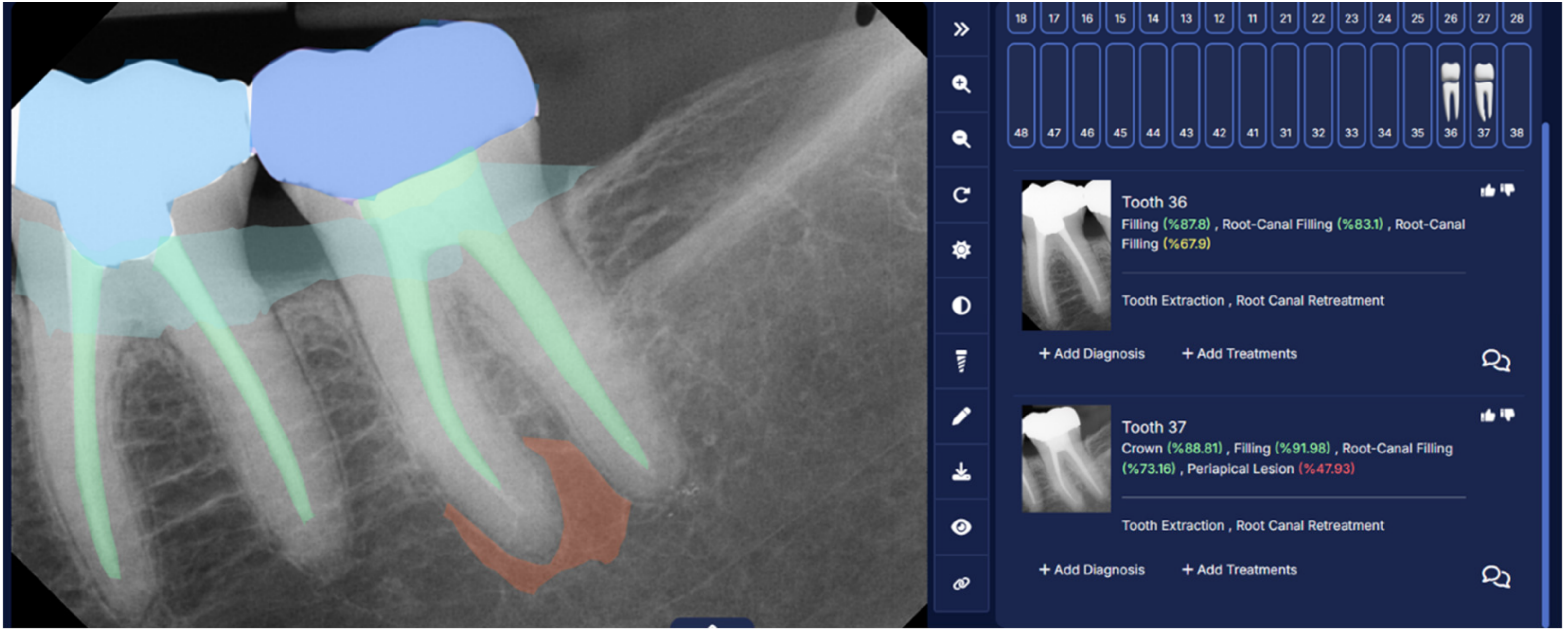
Identification and segmentation of a prosthetic crown and periapical radiolucency on a periapical radiograph. (Courtesy—CranioCatch AI software).
